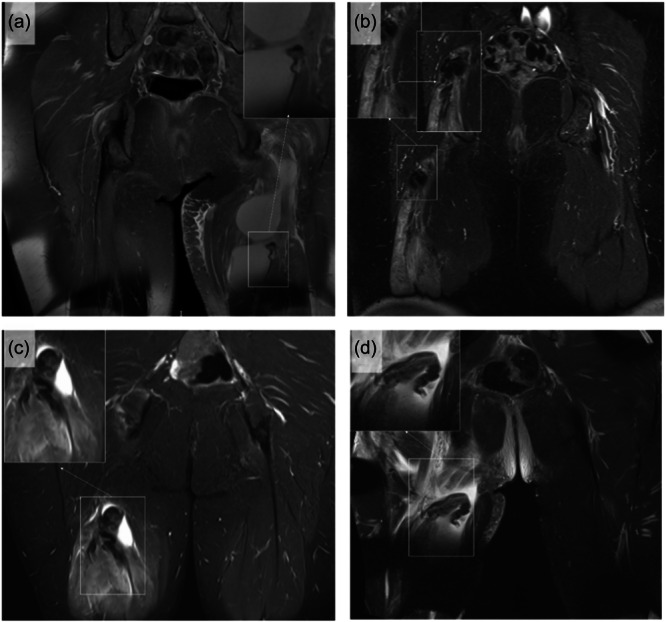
Cobra sign as identified in four patients with complete proximal hamstring avulsion injury. (a) Coronal T2‐weighted MRI of a 53‐year‐old female patient presenting with a sports‐related injury due to a forward fall, showing a complete hamstring avulsion with a Cobra‐like configuration. (b) Coronal T2‐weighted MRI of a 40‐year‐old male patient presenting with a sports‐related injury caused by leg extension and trunk flexion, showing a complete hamstring avulsion with with a Cobra‐like configuration. (c) Coronal T2‐weighted MRI of a 47‐year‐old female patient with a complete hamstring avulsion with a Cobra‐like configuration. (d) Coronal T2‐weighted MRI of a 42‐year‐old male patient presenting showing a complete hamstring avulsion with a Cobra‐like configuration.
